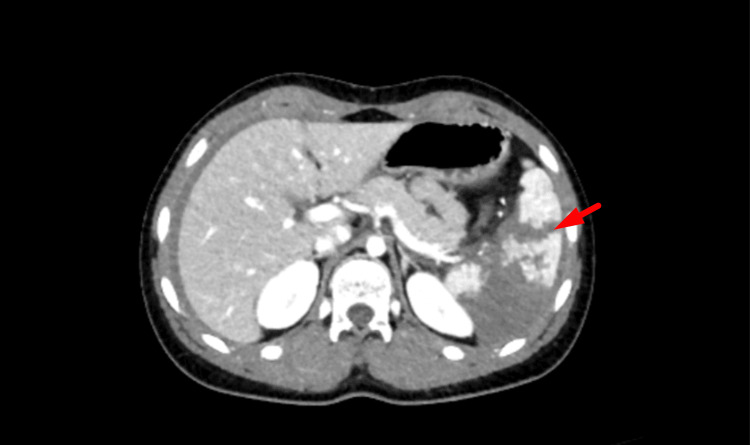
CT cross-section showing rounded intraparenchymal foci consistent with splenic pseudoaneurysms CT: computed tomography
